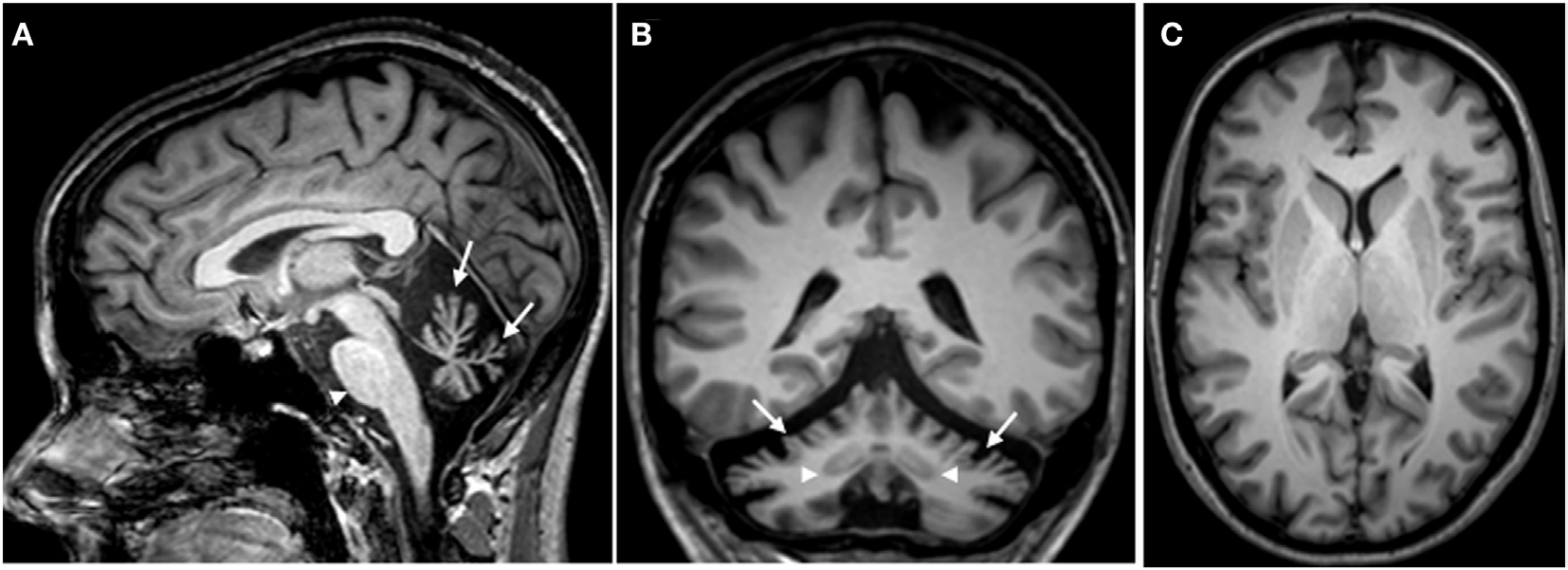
Brain MRI (T1-weighted images) showing a severe atrophy of the cerebellum in the sagittal plane (A). The arrows point to the atrophic vermis, whereas the morphology of the brainstem appears normal (arrowhead). A severe atrophy of the cerebellar cortex is observed in the frontal plane [arrows in panel (B)]. Note that the dentate nuclei are clearly identified on both sides (arrowheads). Striatum, thalamus, subcortical white matter, and cerebral cortex appear morphologically normal on both sides [axial image in panel (C)].
